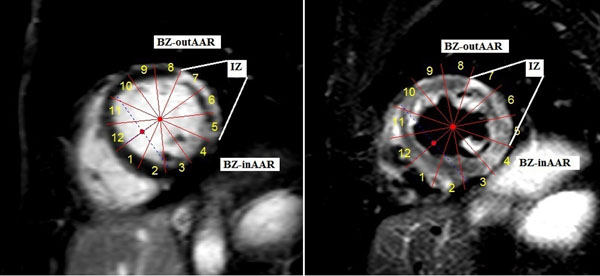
The left figure shows the late gadolinium enhancement midventricular short axis slice of an acute phase MI, with segments 5-7 defined as IZ (including microvascular obstruction), segments 4 and 8 as BZ and the remaining segments as RM. The right figure shows the corresponding T2-weighted image, with segment 4 defined as BZ-inAAR, and segment 8 as BZ-outAAR.
